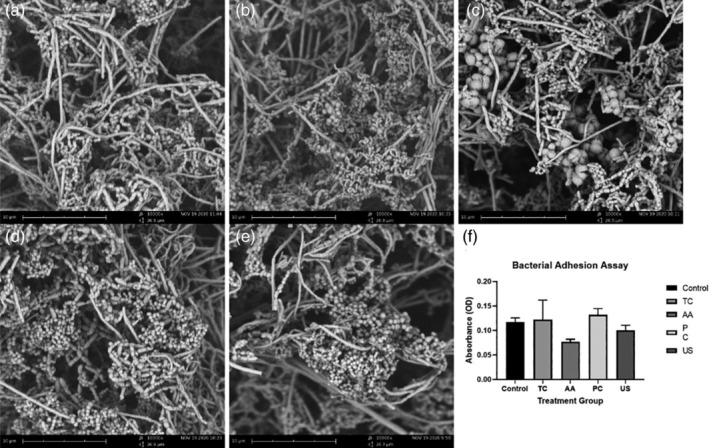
Formation of biofilms on acid‐etched zirconia surfaces is not affected by instrumentation. Biofilms of salivary bacteria were established on zirconia discs as described in the materials and methods. After 48 h incubation, non‐adhered bacteria were washed away and attached bacteria visualized by SEM (a–e). Spherical‐shaped bacterial cells intertwined within a dense network of extracellular matrix are visible. Representative images (10,000× magnification) are shown for each instrument used. (a) Untreated (b) titanium curette (TC); (c) air abrasive device (AA); (d) plastic curette (PC) (e) ultrasonic scaler (US). In separate experiments attached bacteria were dislodged and numbers estimated by measurement at OD600 (f). Results are presented as mean ± standard error, (three discs per group)
